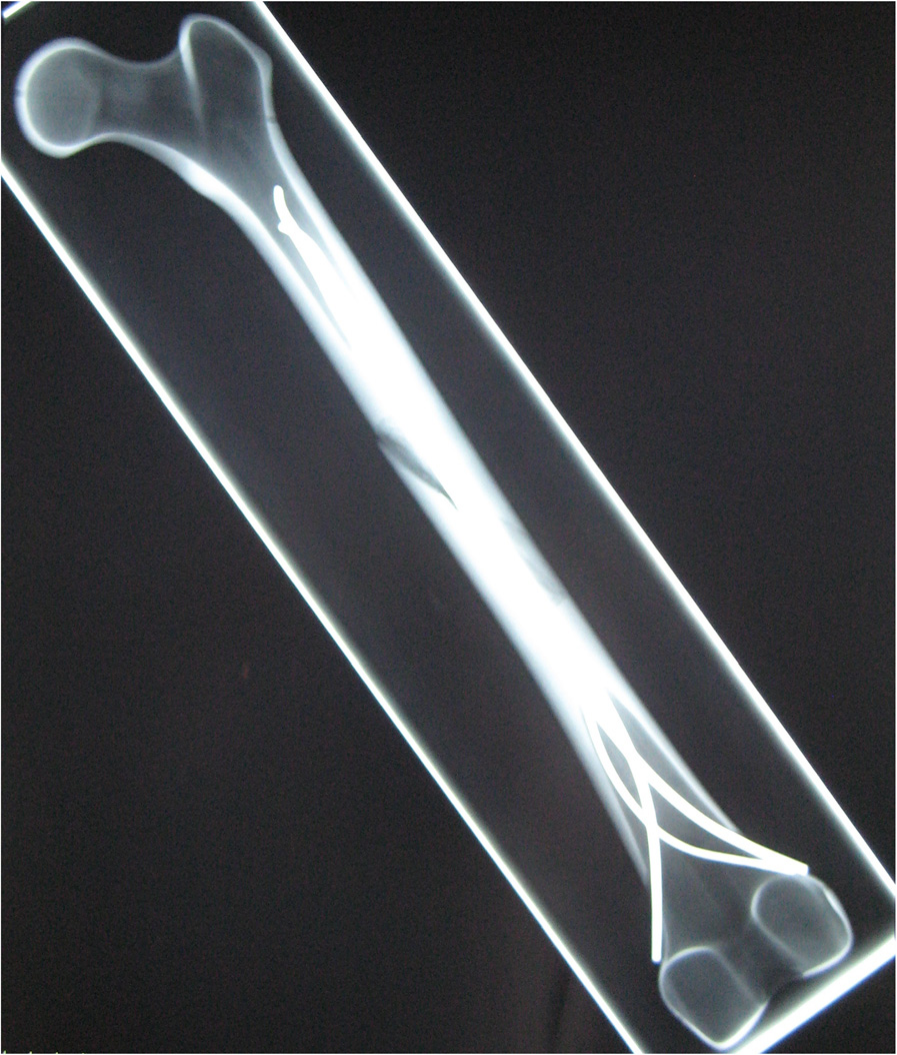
Radiograph (anterior-posterior view) of a 3CL femoral model with retrograde intramedullary nailing, showing two 3.5 mm titanium nails implanted in 2C shaped configuration and one additional 2.5 mm titanium nail implanted antero-lateral.
